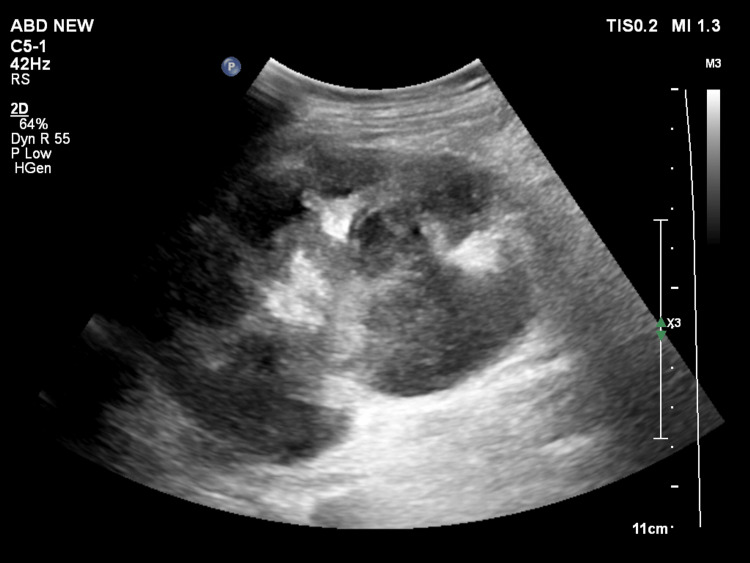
Repeat ultrasonography showing the reniform shape of the kidney after resolution of the abscess cavity with few pockets of intrarenal hypoechoic collection.
